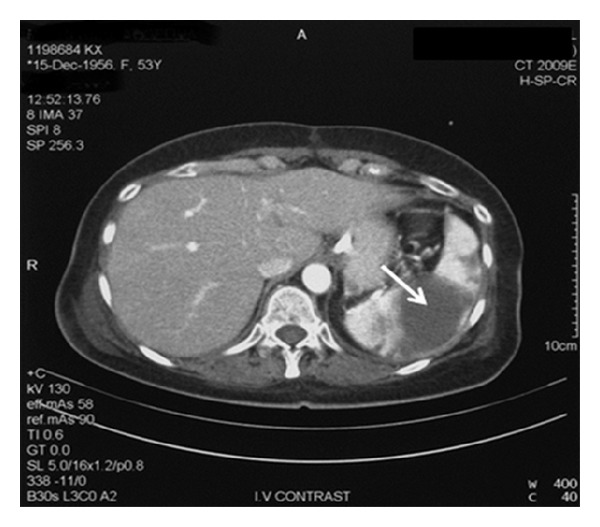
Abdominal CT with splenic infarcts.
